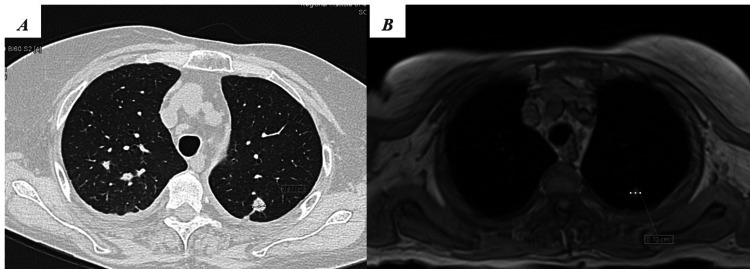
(A) Axial view of HRCT of the thorax demonstrating a well-defined pulmonary nodule in the LUL apico-posterior segment. (B) T1-VIBE MRI sequence; the nodule is clearly depicted as hyperintense in the same segment, confirming high sensitivity for this size range (>7 mm). HRCT = high-resolution computed tomography; LUL = left upper lobe; T1-VIBE = volumetric interpolated breath-hold examination; MRI = magnetic resonance imaging
